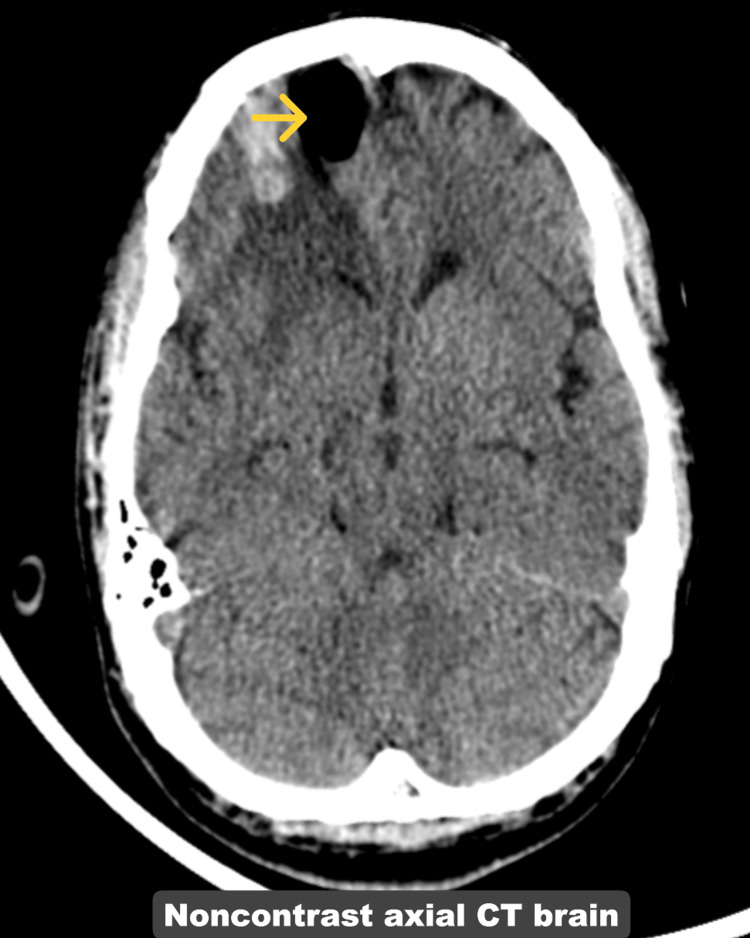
Day 14 non-contrast axial CT brain showing new basifrontal air collection (intracerebral pneumatocele) The yellow arrow shows a new air collection (intracerebral pneumatocele) in the right basifrontal region.
